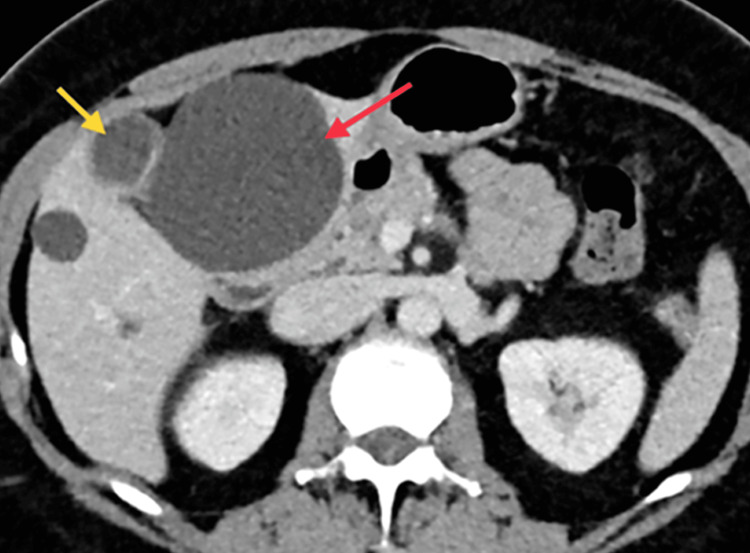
Transverse CT image cross-section of the abdomen displayed a 7.5-cm hepatic cyst (red arrow) and compressed gallbladder (yellow arrow)
